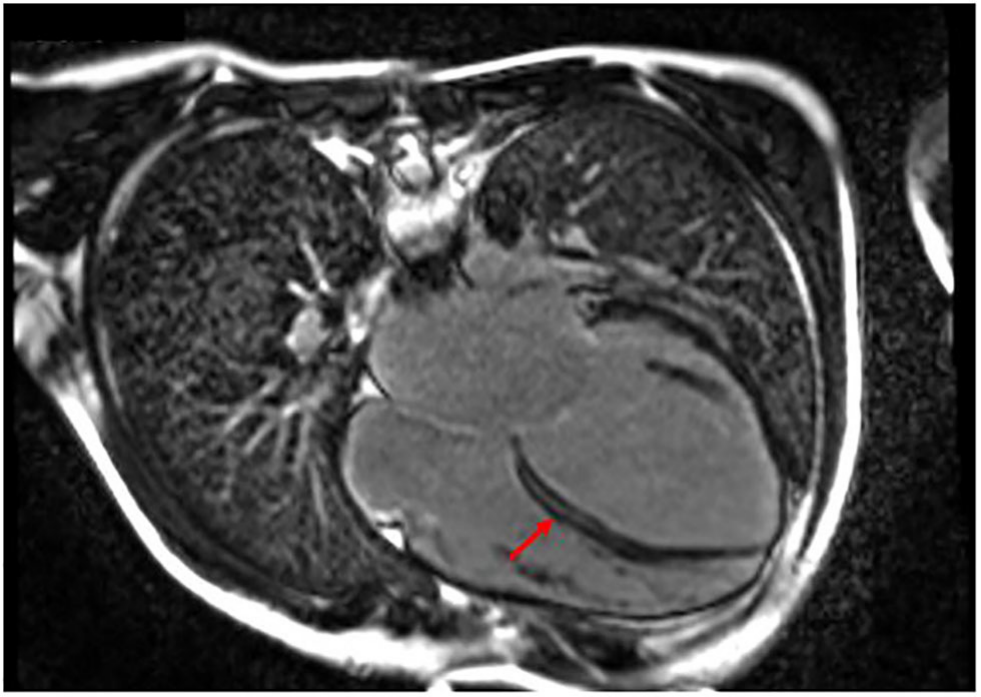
Patient 3 focal image of cardiac MRI in a 4-chamber view showing LGE with midwall fibrosis of the interventricular septum at medium–basal level of the basal inferior wall. The red arrow indicates the areas with major involvement of LGE (late gadolinium enhancement).
